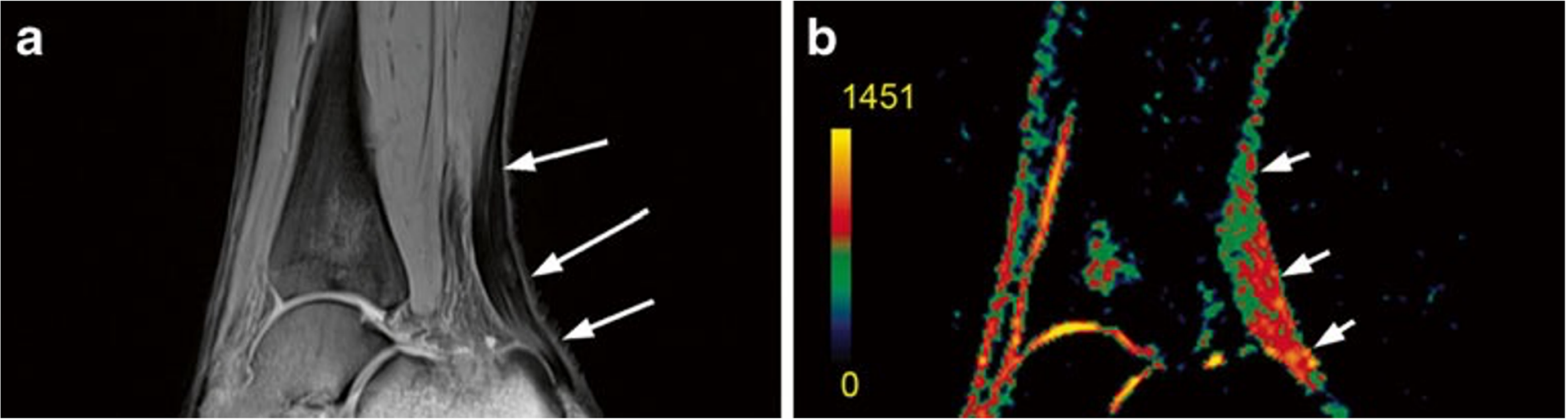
MRI of a 46-year-old patient with chronic Achilles tendinopathy. a Proton-density-weighted two-dimensional turbo spin-echo image depicts centres of each region of interest (arrows). b Corresponding colour-coded three-dimensional gradient-echo sodium image depicts Achilles tendon sodium signal (arrows), which is obviously higher than that in the healthy volunteer. The sodium signal increase was observed in the whole tendon, and not only in regions with clinical findings (middle region in this case). All patients had focal thickening of the Achilles tendon without fluid-like signal intensity increase on proton-density–weighted two-dimensional turbo spin-echo images. Colour scale represents sodium signal intensity values. Reprinted with permission from: Juras et al., Radiology 2012 (reference [63])
